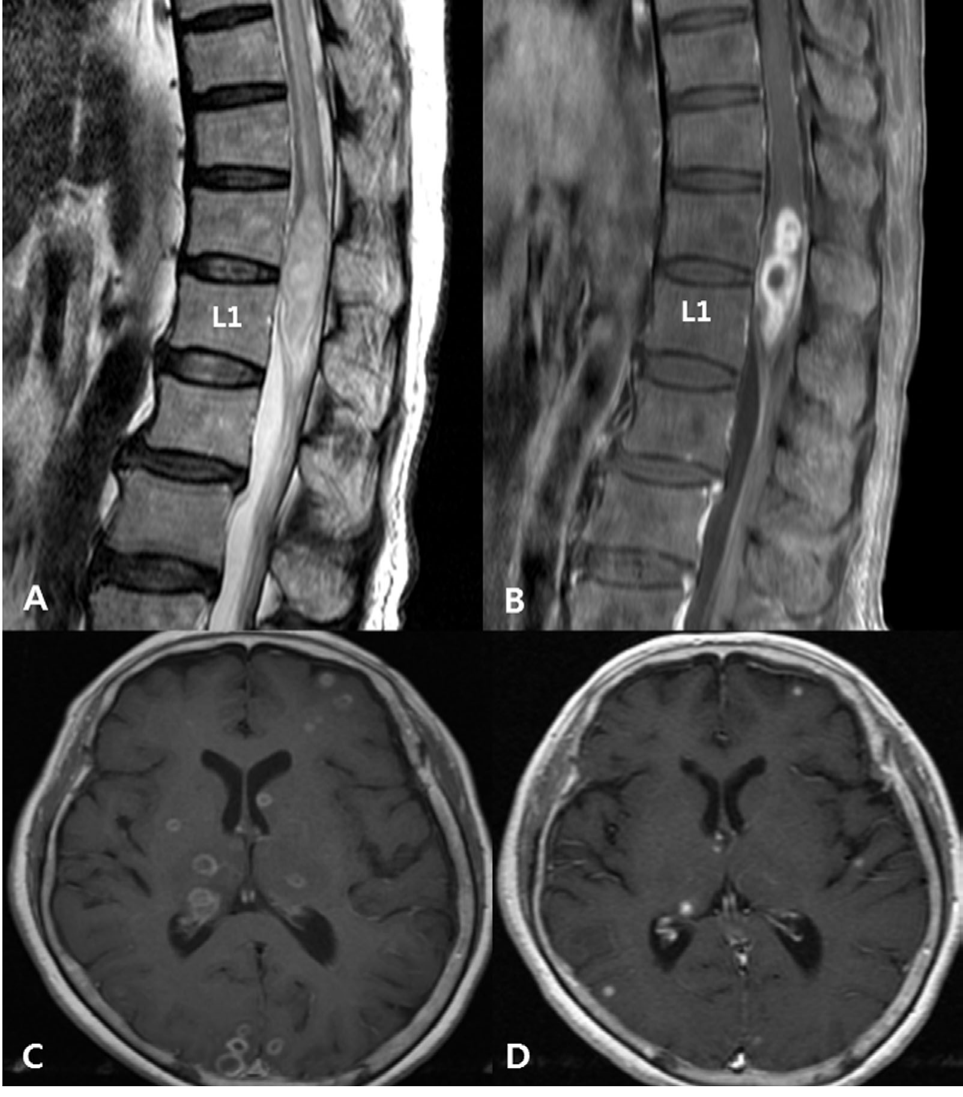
Disseminated tuberculosis in a 72-year-old woman with pain in both lower extremities with underlying active pulmonary tuberculosis. (A) Spinal magnetic resonance (MR) T2-weighted images show intermediate to slightly high signal intensities and cord edema at the T7–L1 level. (B) Post-contrast T1-weighted images with fat suppression, taken at the same level as the images in panel (A), show rim enhancement at the intramedullary portion of the conus medullaris, suggesting a tuberculous abscess. (C) Initial post-contrast T1-weighted brain MR image shows multiple tuberculomas with rim enhancing pattern. (D) Follow-up MR image shows regression of tuberculomas after a 1-month anti-tuberculosis treatment.
